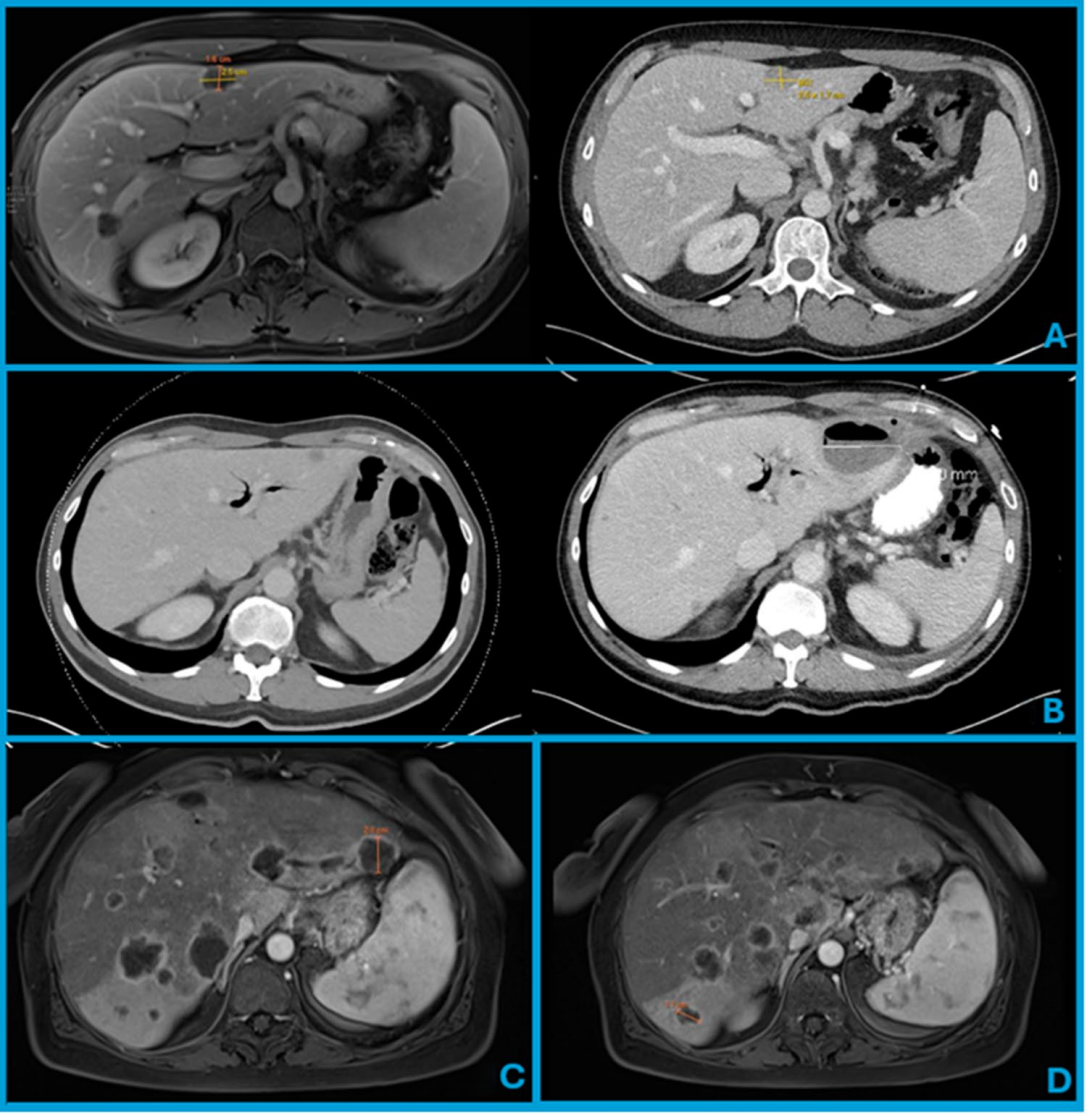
A Sequential imaging studies two months apart showing a non-progressing metastatic liver lesion secondary to treatment response that was found by laparoscopic biopsy to be necrotic and nonviable. The planned liver resection was aborted. B CT scan showing left-sided pneumobilia due to the presence of a biliary stent and a segment II liver lesion that was resected for TIL (left). The patient subsequently developed an abscess at the resection site requiring placement of a percutaneous drain (right). C Magnetic resonance imaging showing a metastatic liver lesion in segment II/III with a dilated proximal bile duct. D Magnetic resonance imaging showing a metastatic liver lesion in segment VI with a regional perfusion defect due to vascular involvement of proximal metastases
